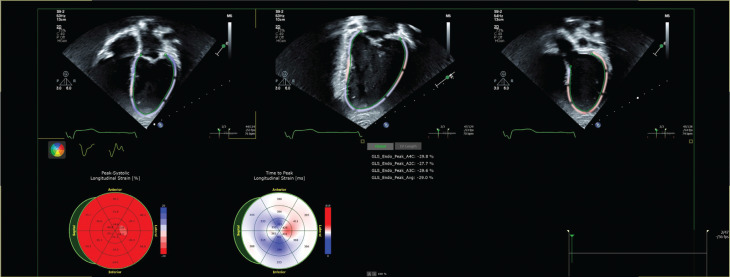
Global longitudinal strain imaging for the apical two-, three-, and four-chamber views.
